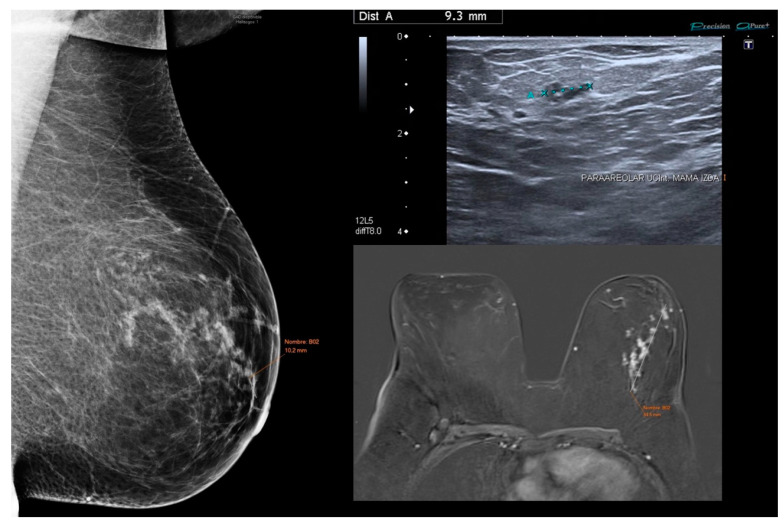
Low-grade DCIS case demonstrating variation in tumor size estimation across imaging modalities. Mammography, ultrasound, and MRI show differing lesion dimensions (10.2 mm, 9.3 mm, and 84.5 mm, respectively).
